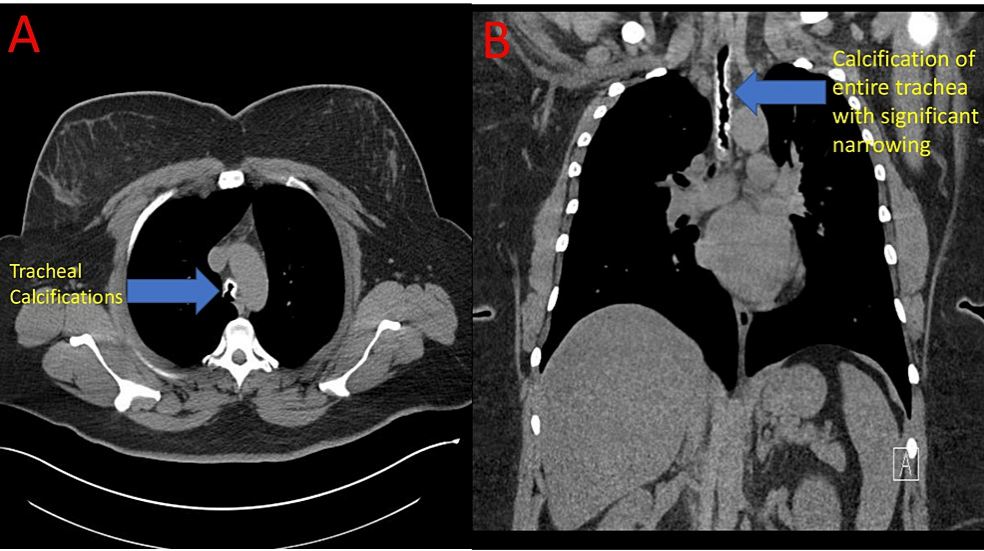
CT chest (A - axial view, B - coronal view) Inspiratory images from the CT scan of the chest ( A, B). Image A demonstrates tracheal calcifications (blue arrow). Image B demonstrates calcifications of the entire trachea with resultant severe tracheal narrowing (blue arrow).
